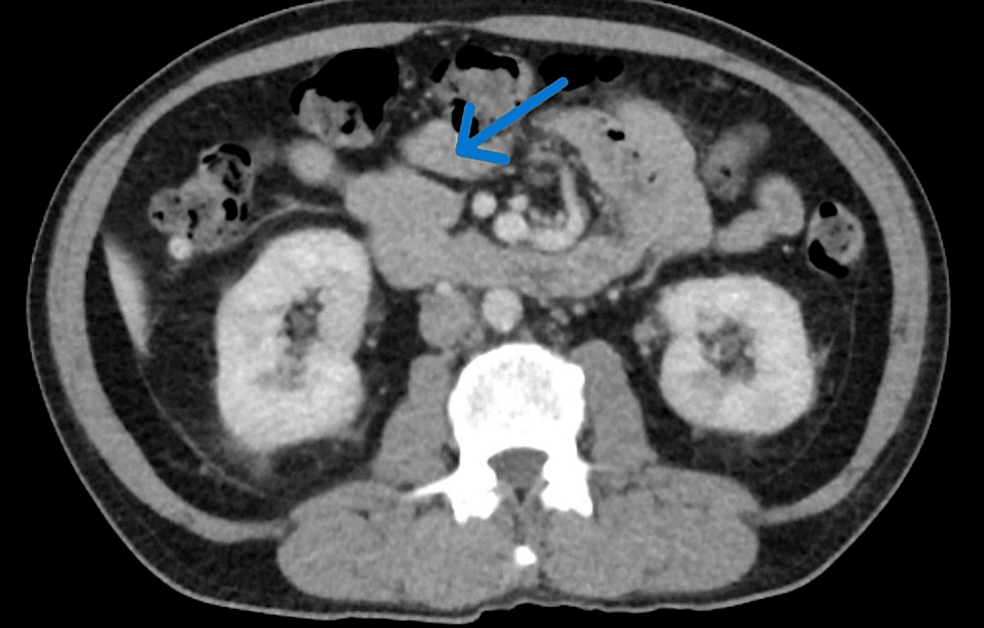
MRI showing the enlarged pancreatic mass that was in question
